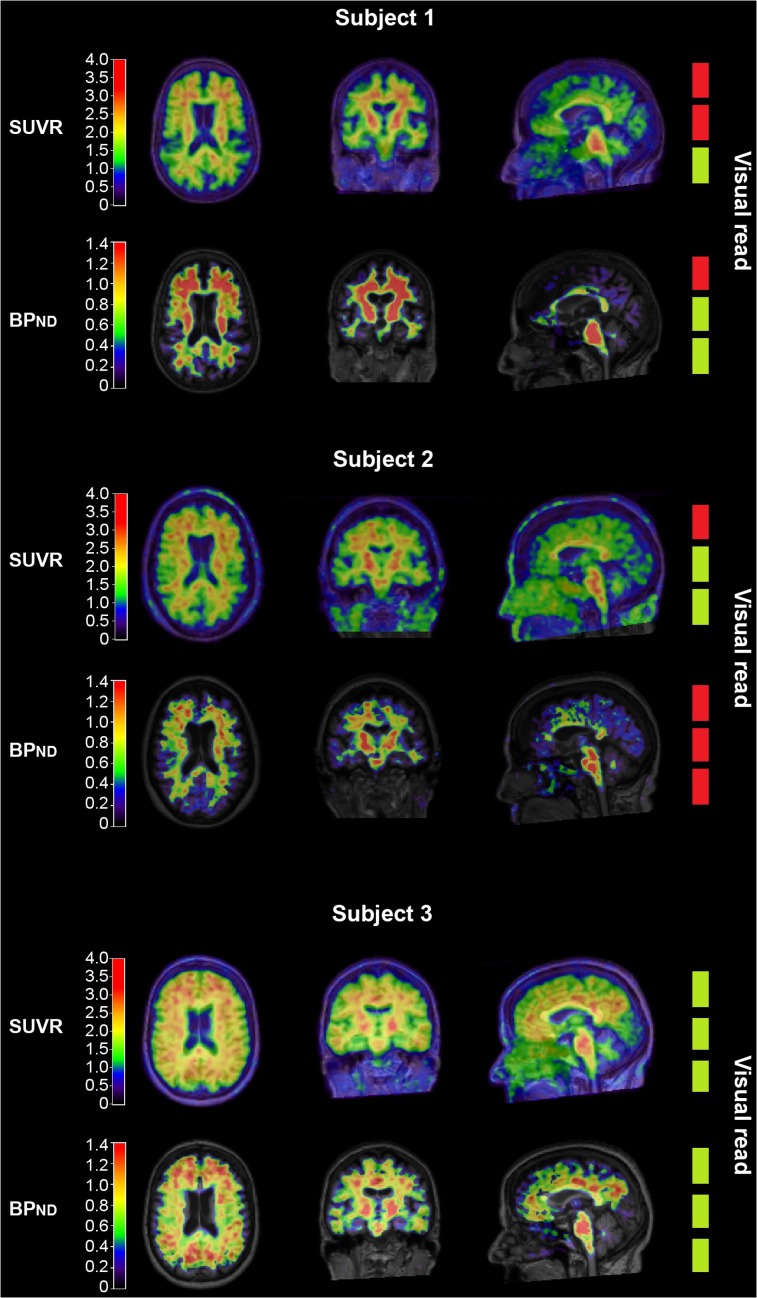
Examples of SUVr and BP_ND_
